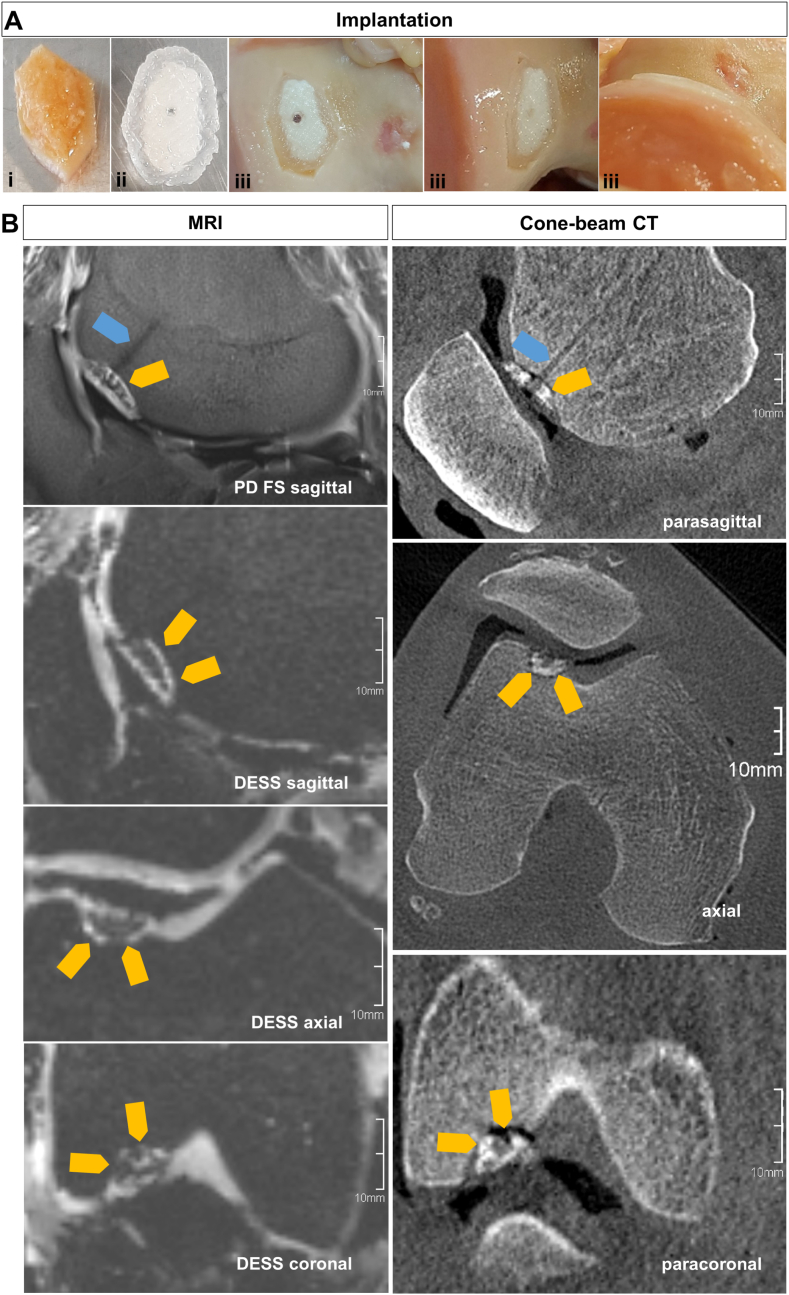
Implantation (A) and post-implantation imaging (B) exemplarily shown for defect No. 5. A: Osteochondral fragment (i) removed prior to implantation, the implant structure before (ii) and after (iii) implantation. Clinical images showing the inserted implant fixed with a SmartNail® through a drilled central hole. B: Three-dimensional reformations along the implant in high-resolution MRI und CT. The orange arrows mark the defect filled with the implant, the blue arrows the channel with the SmartNail®. (For interpretation of the references to colour in this figure legend, the reader is referred to the Web version of this article.)
